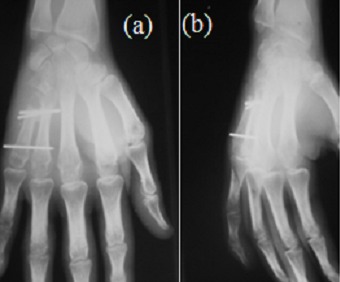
Radiographie de la main droite (A: incidence de face, B: incidence oblique) résection arthroplastique de la base du M5 et arthrodèse métaphyso-diaphysaire des deux derniers métacarpiens stabilisée par embrochage transversal
